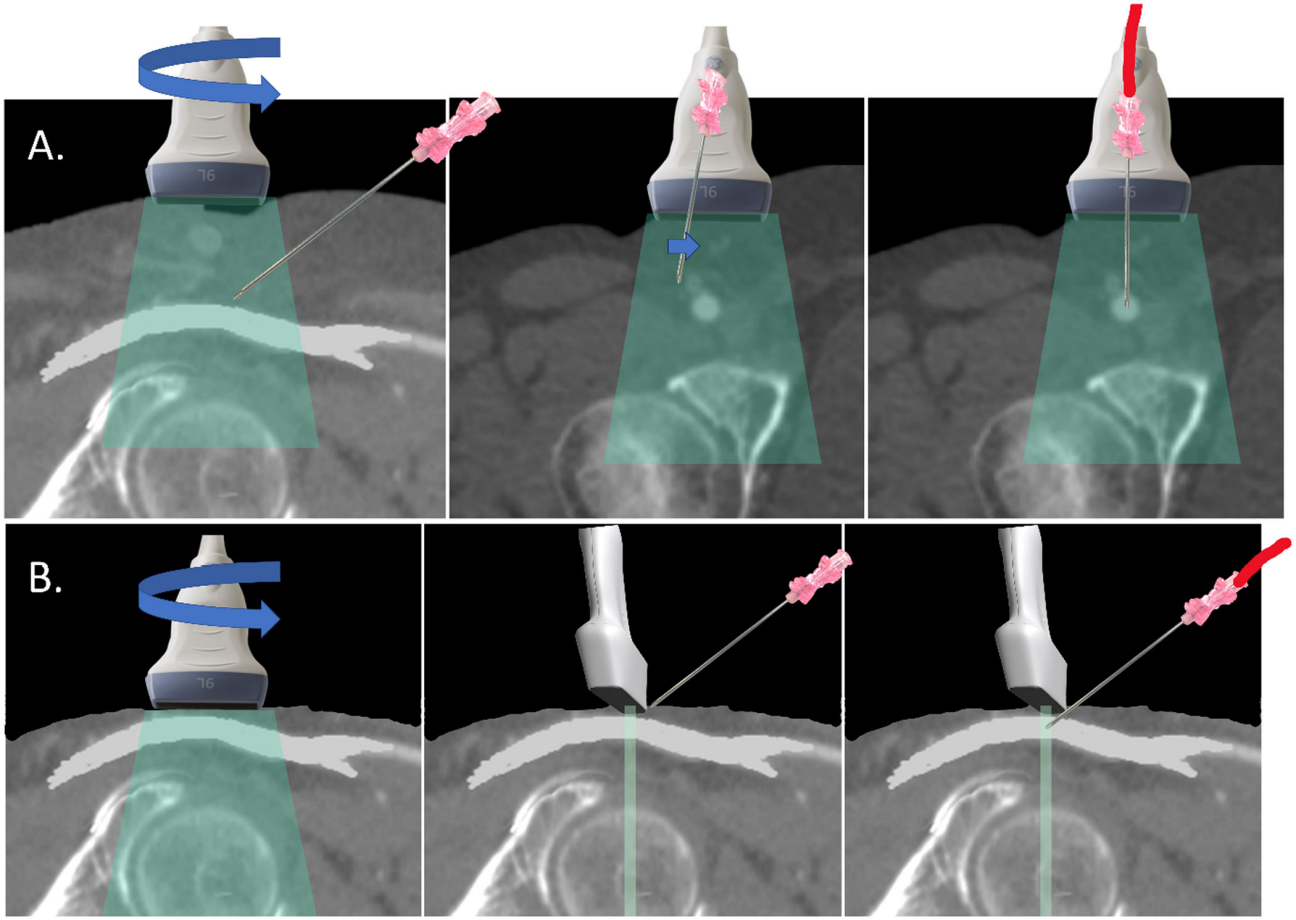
Alternative techniques for ultrasound‐guided CFA puncture. (A) When the operator struggles to maintain alignment of the needle tip with the ultrasound beam as it nears the CFA—resulting in a loss of needle visualization on the screen—they can rotate the transducer to a transverse view. This adjustment helps ensure a central puncture of the vessel. (B) In patients with very thin subcutaneous tissue (less than 1–2 cm), where there is insufficient space to visualize and maneuver the needle in the longitudinal ultrasonographic view, it is preferable to place the ultrasound probe directly over the chosen puncture site in a transverse orientation to the common CFA. The needle should then be advanced perpendicularly at a 45° angle, targeting the center of the artery. [Color figure can be viewed at wileyonlinelibrary.com]
